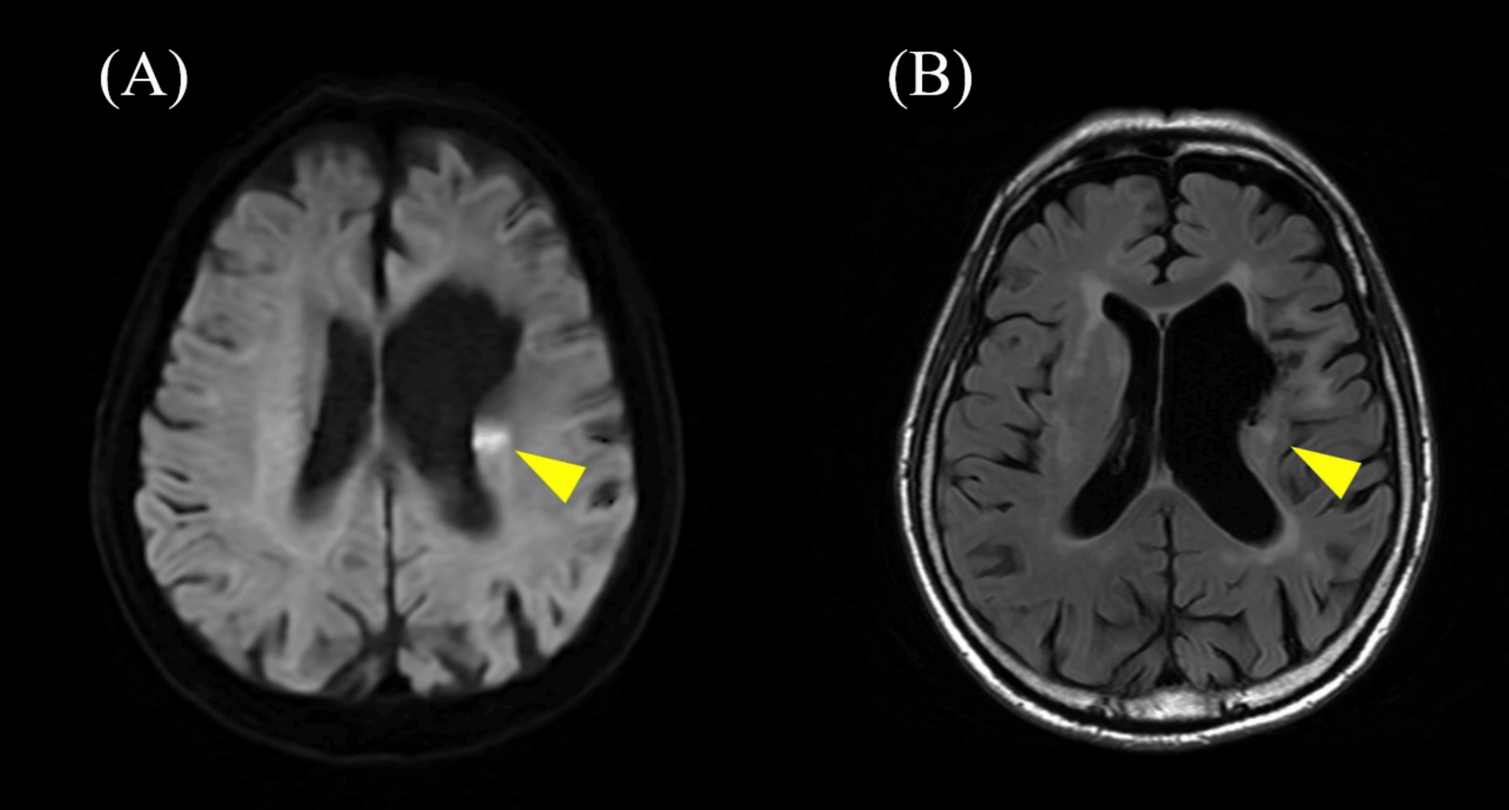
Brain magnetic resonance imaging (MRI) at stroke onset (obtained at a previous hospital) at age 76. Brain MRI showing a hyperintensity area in the left corona radiata (arrowhead): (A) diffusion-weighted imaging (DWI); (B) T2-fluid attenuated inversion recovery imaging (T2-FLAIR).
